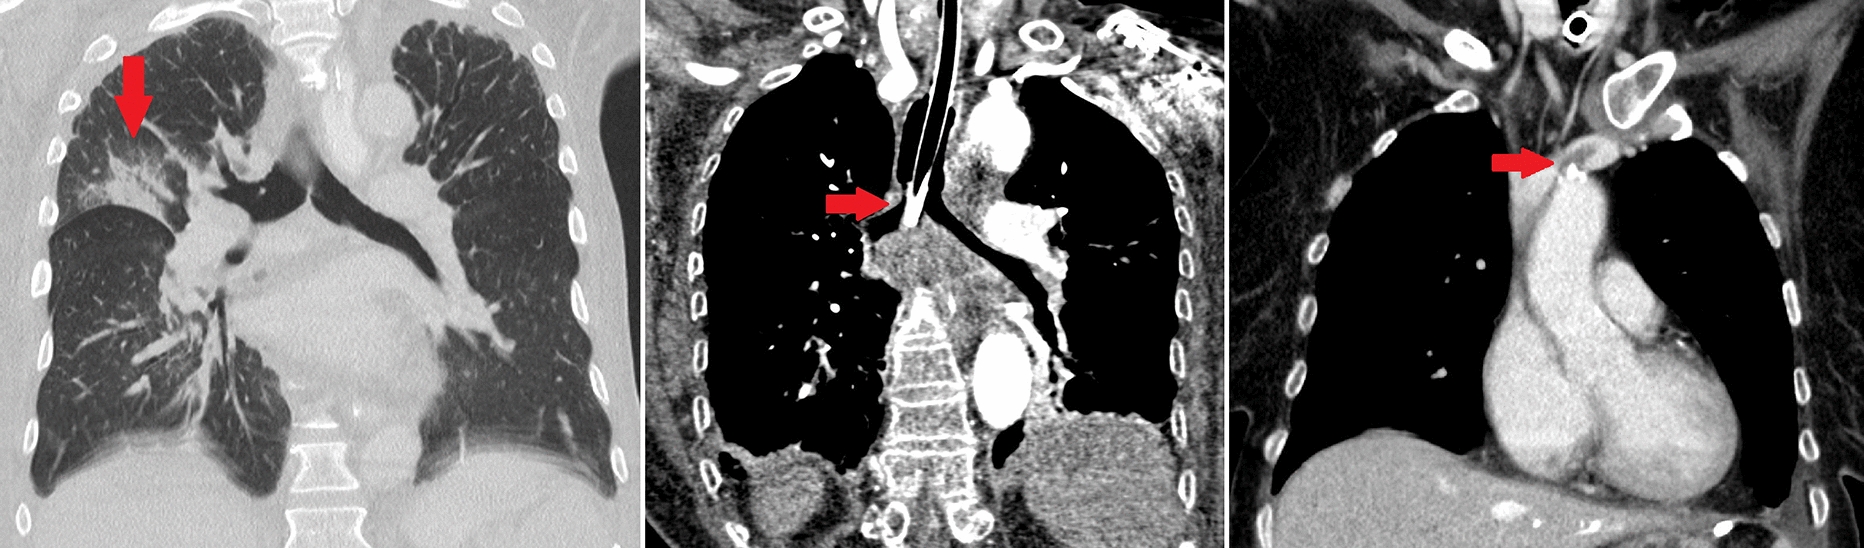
Examples of imaging findings on thoracic CT above the diaphragmatic dome. Left: Coronal contrast enhanced CT showing pneumonic infiltrate in the right upper lobe. Middle: Coronal contrast enhanced CT showing endotracheal tube malposition in the right main bronchus. Right: Coronal contrast enhanced CT showing catheter-associated thrombosis in the left brachiocephalic vein
